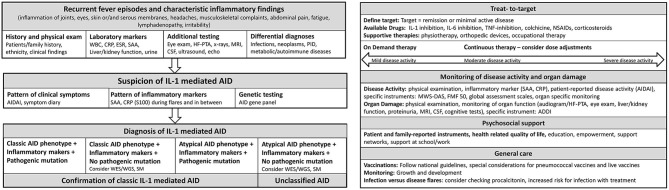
Flow sheet diagnostic steps and management of AID. AID, autoinflammatory disease; WBC, whole blood count; CRP, c reactive protein; ESR, erythrocyte sedimentation rate; SAA, Serum Amyloid A; HF-PTA, high frequency pure tone audiogram; MRI, magnet resonance imaging; CSF, cerebrospinal fluid; PID, primary immune deficiency; IL, Interleukin; AIDAI, Autoinflammatory Disease Activity Index; S100, S 100 proteins; WES, whole exome sequencing; WGS, whole genome sequencing; SM, somatic mosaicism; TNF, Tumor necrosis factor; NSAIDs, Nonsteroidal anti-inflammatory drugs; MWS-DAS, Muckle Wells Syndrome Disease activity score; FMF 50, Familial Mediterranean Fever 50 Score; ADDI, Autoinflammatory Disease Damage Index.
